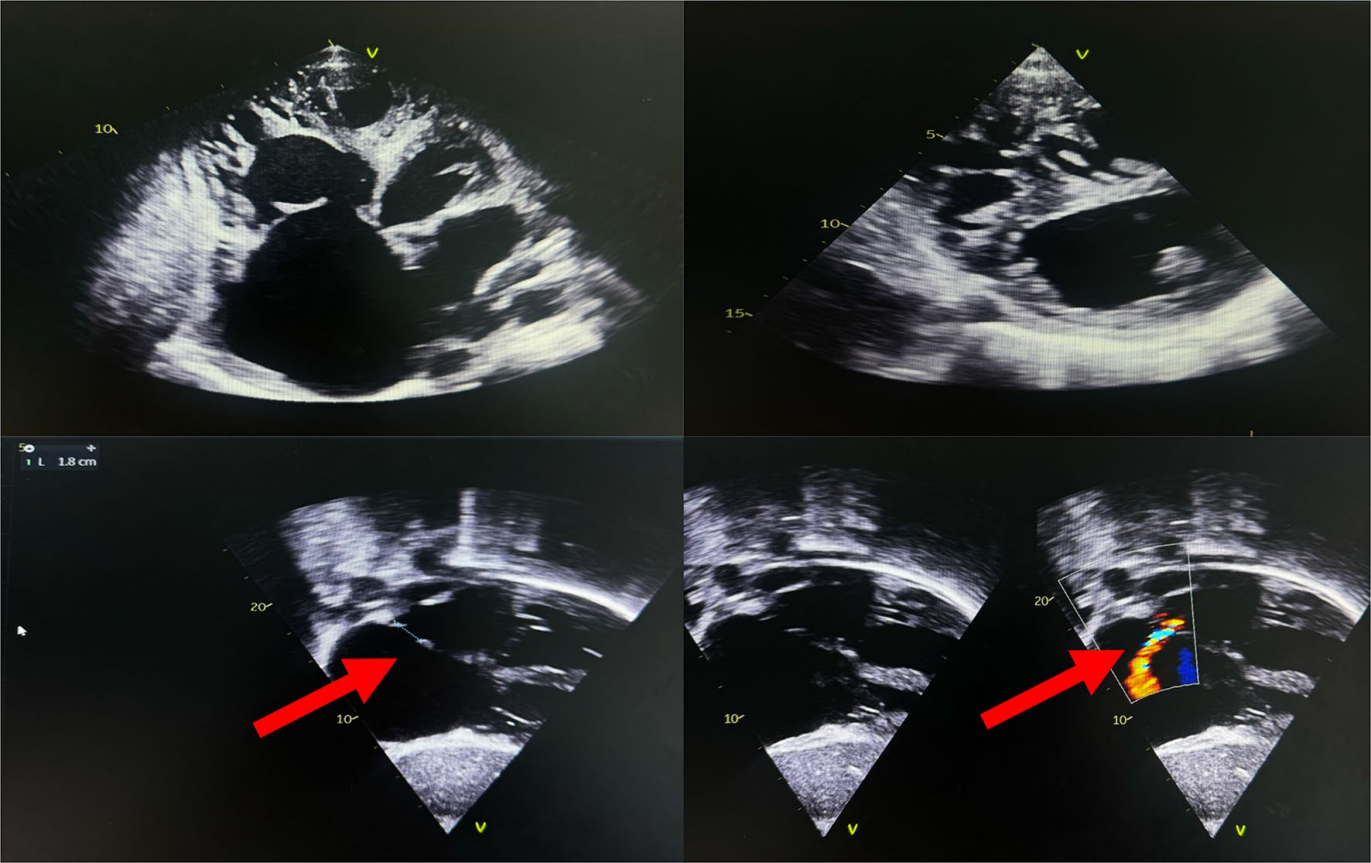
Transthoracic echocardiography showing interatrial communication ostium secondum (red arrows)
